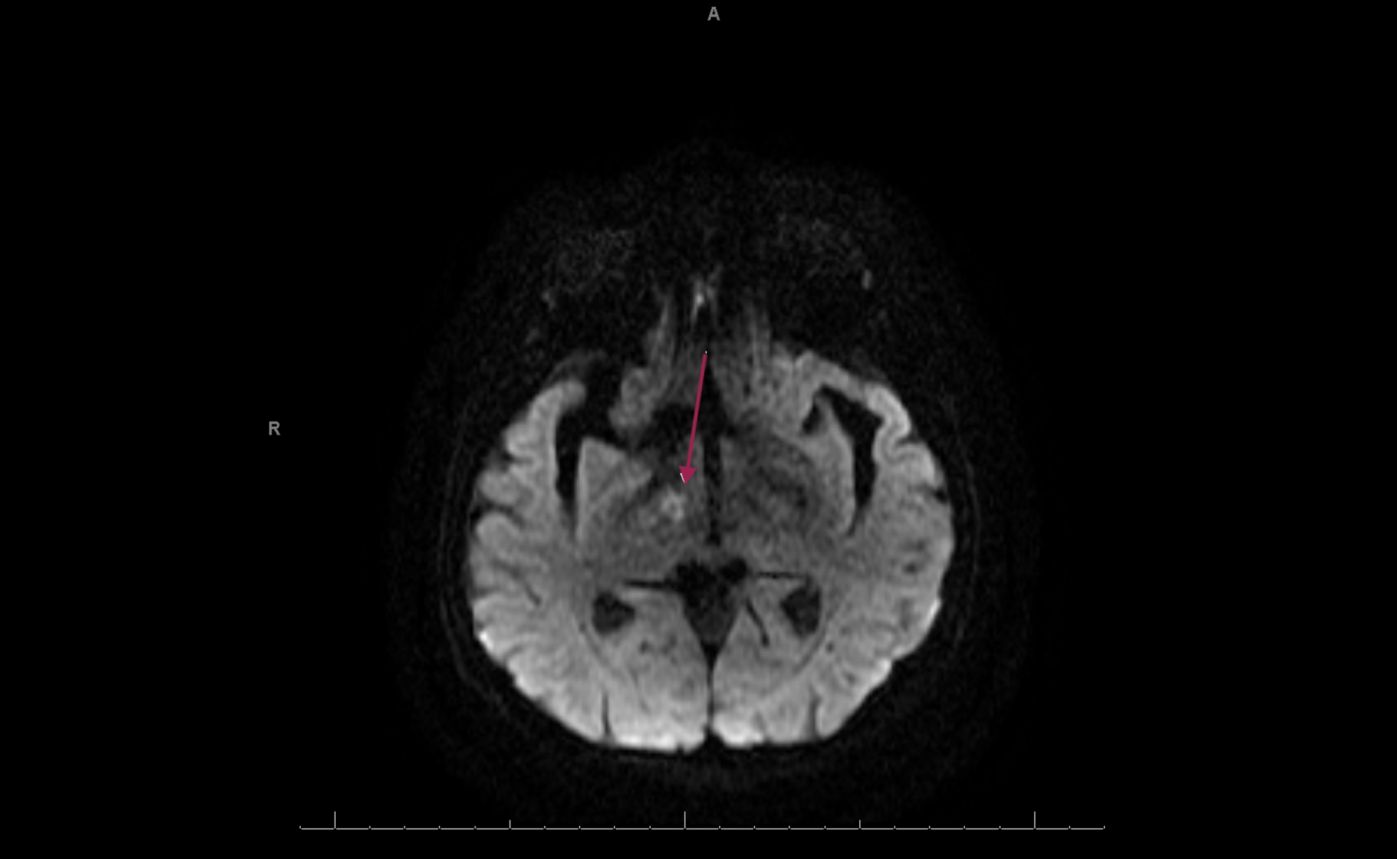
MRI brain axial DWI of acute to subacute right thalamic lacunar infarct. DWI, diffusion weighted imaging
